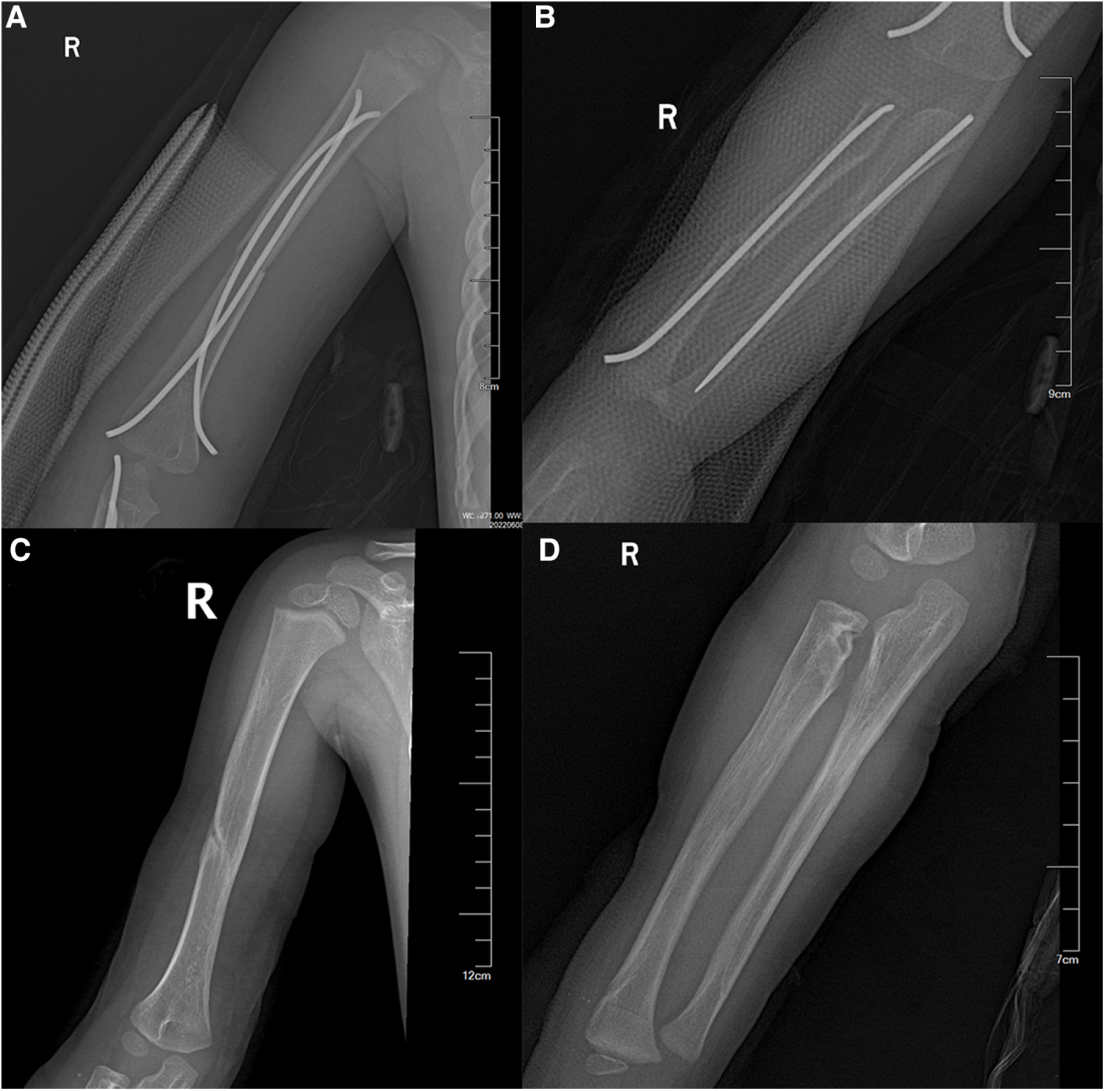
x-ray images of the child one day after surgery (A,B); x-ray images of the child after one year, following the removal of the internal fixation, showing avascular necrosis of the radial head (C,D).
